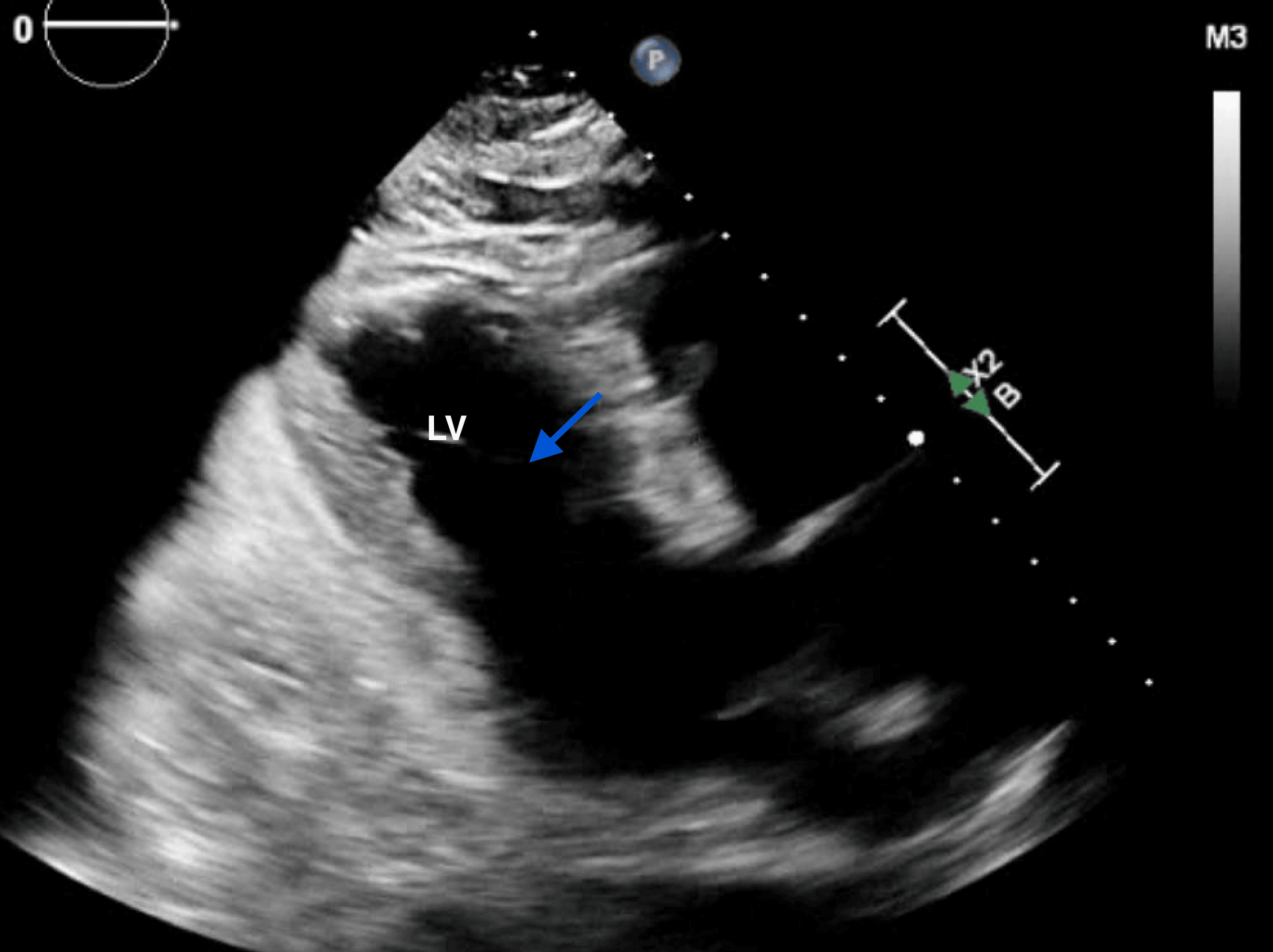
Transthoracic echocardiogram (parasternal long-axis view) at maximal left ventricular contraction, showing reduced ejection fraction and elevated end-systolic volume. The blue arrow denotes increased end-systolic volume. LV, left ventricle
